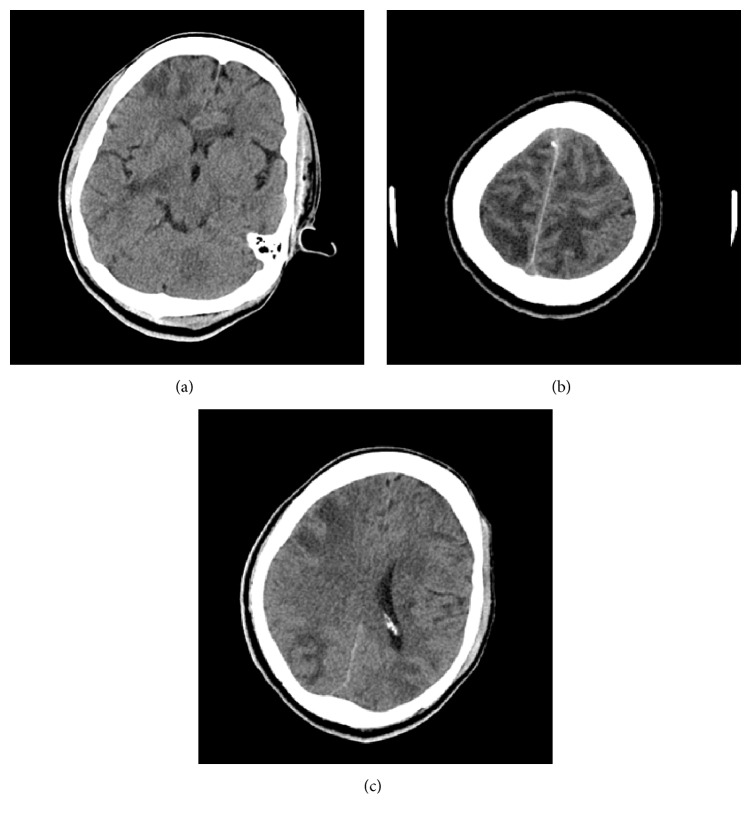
CT Head-Axial images demonstrate extensive multifocal areas of white matter hypoattenuation/edema.
